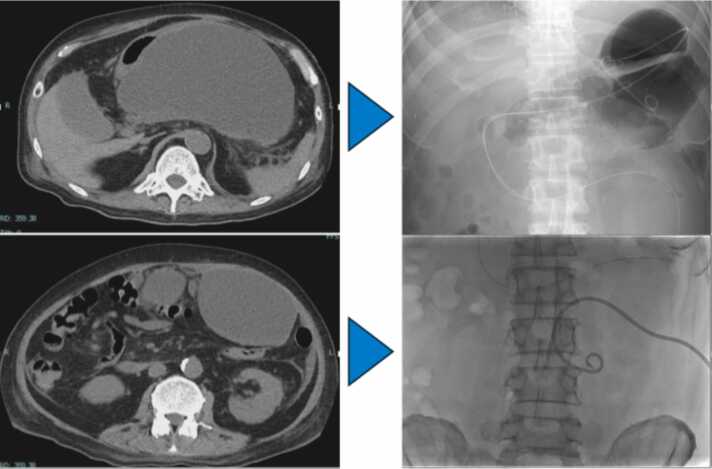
Abdominal Computed tomography (CT) and fluoroscopic images demonstrate multiple large cystic lesions in the abdominal cavity, including dorsal to the stomach and near the anterior abdominal wall. These lesions were treated using transgastric and percutaneous drainage.
